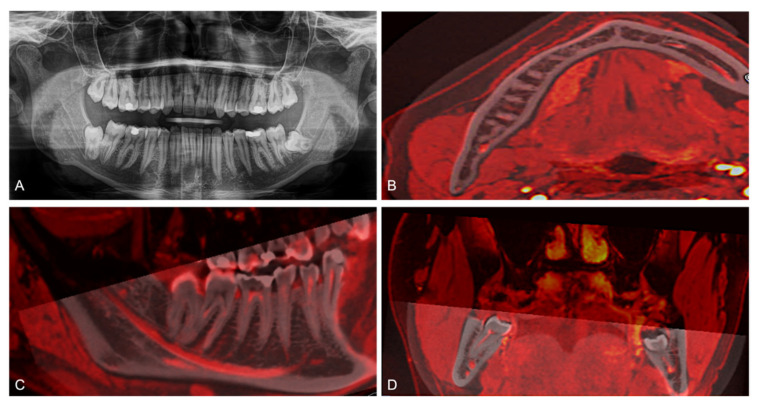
(A) Two-dimensional panoramic radiography (PAN) of a study participant, CBCT and MRI scans of a study participant were transferred to OnDemand3D and fused using the “automatic registration” function at an equal slice thickness of 0.5 mm. The MRI scan was labeled red, and conventional grayscale values displayed the CBCT scan. Axial (B), sagittal (C), and coronal (D) fused CBCT/MRI images are visualized.
